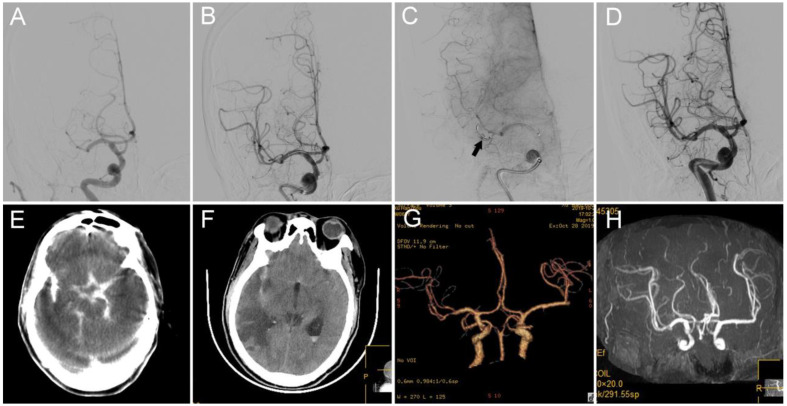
(A) DSA shows occlusion of the M1 segment of the right MCA. (B) The microcatheter advanced through the occlusion site, and a Solitaire 4–20 mm was unsheathed at the occlusion site. (C) Angiography showed contrast extravasation from the inferior trunk of the MCA (indicated by the black arrow). (D) No contrast extravasation after injection of 200 U thrombin via the microcatheter. mTICI 3 reperfusion was achieved when the microcatheter was re-advanced into the inferior trunk of the MCA over the microwire and retrieved back into the proximal segment of the MCA while the microwire remained in place. (E) Hyperdensity within the Sylvian fissure and ventricle was observed on C-arm CT performed during the procedure. (F,G) Hyperdense area dismissed on follow-up CT. (G,H) Patency of the culprit artery was observed on follow-up CTA and MRA. DSA: digital subtraction angiography; MCA, middle cerebral artery; mTICI: modified thrombolysis in cerebral infarction; CTA: computed tomography angiography.
